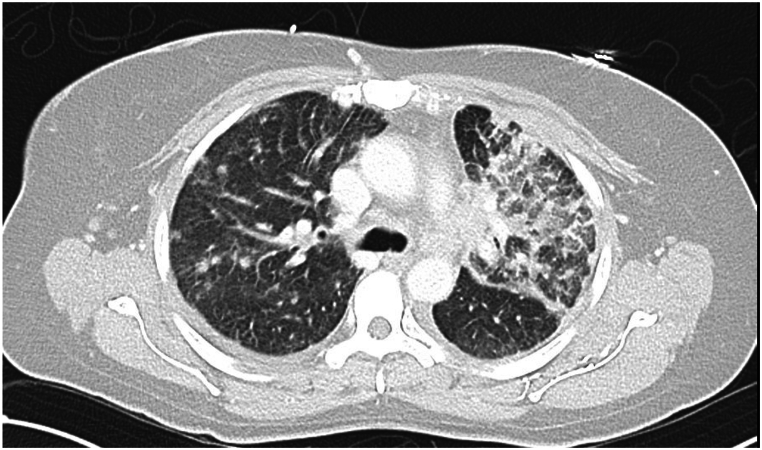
CT Chest showing bilateral lung infiltrates, predominantly in the left upper lobe.
